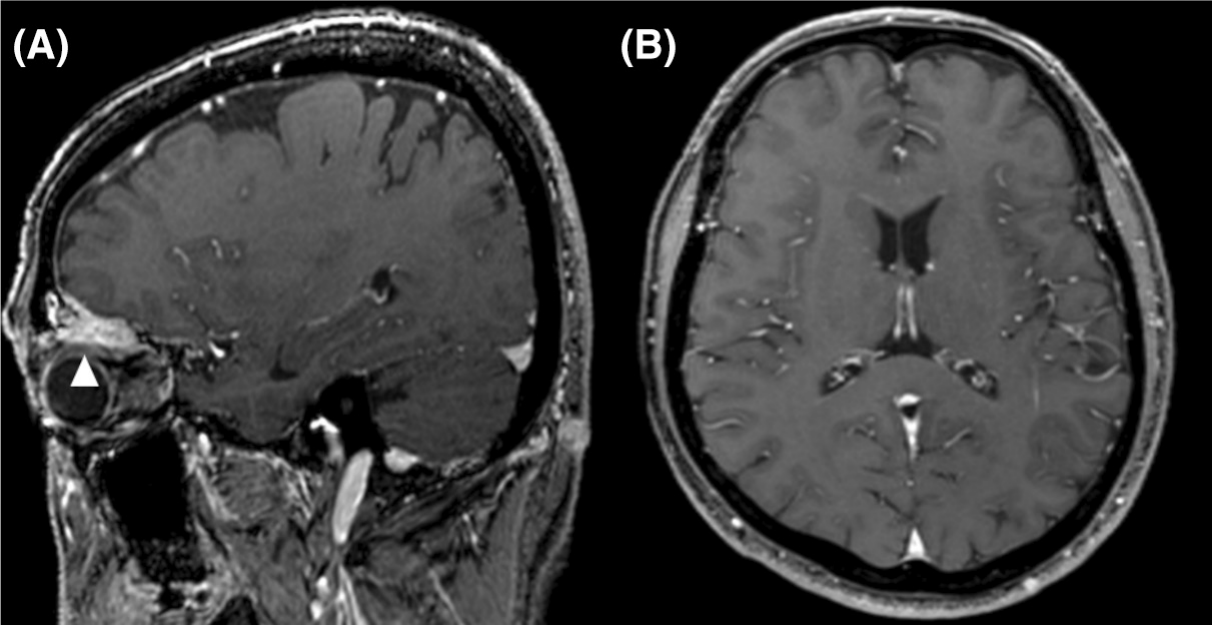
Gadolinium‐enhanced head magnetic resonance imaging indicative of skull base bone metastasis without evidence of hematoma and brain metastasis (A, B).
